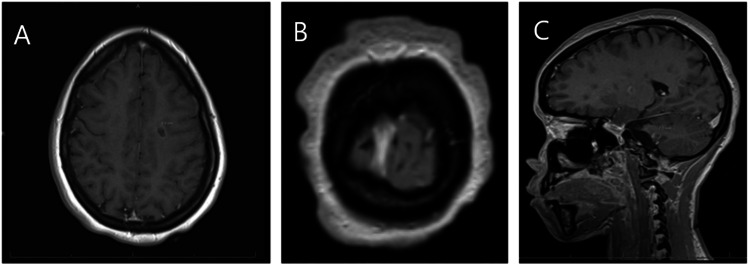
Brain MRI demonstrating multifocal enhancing supratentorial and infratentorial demyelinating lesions consistent with active multiple sclerosis. Brain MRI. (A) Axial T1-weighted post-contrast image demonstrating an enhancing demyelinating plaque. (B) Additional axial brain MRI slice highlighting a focal lesion. (C) Sagittal T1-weighted post-contrast image demonstrating an enhancing infratentorial plaque (measurement shown), consistent with active demyelination.
